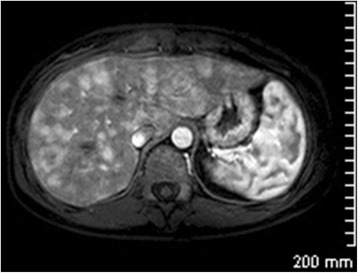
Pre-treatment axial T1-weighted contrast-enhanced MRI showing innumerable avidly enhancing, bilobar hepatic metastases
